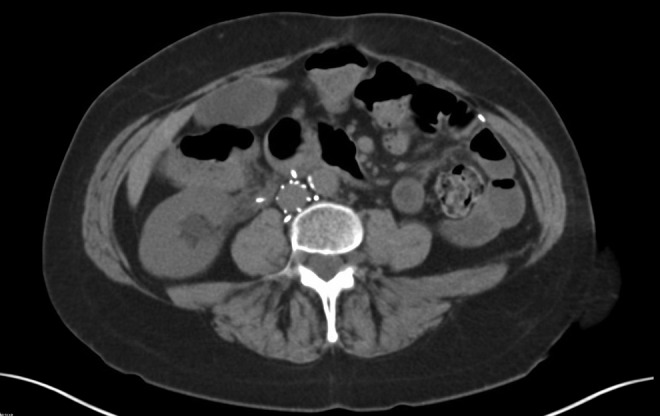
Axial cut CT showing erosion of multiple IVC filter limbs, including limb projecting into lumen of right ureter with proximal hydroureteronephrosis.
